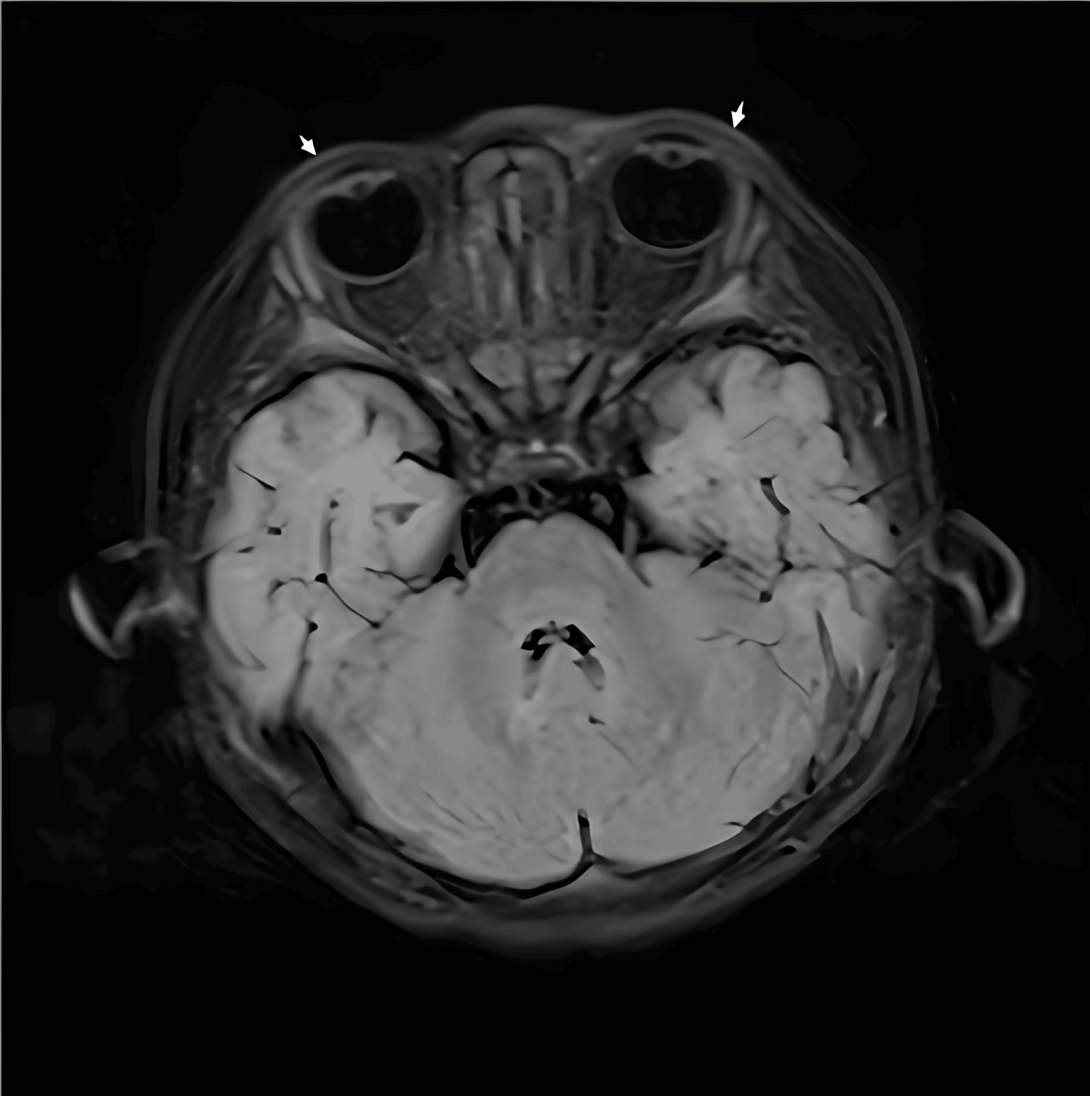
MRI of the head and orbits at the time of admission The figure presents an orbital MRI utilizing T2-weighted FLAIR imaging. The horizontal scan demonstrated the presence of bilateral, localized edema of the eyelids (white arrows). MRI: magnetic resonance imaging, FLAIR: fluid-attenuated inversion recovery
